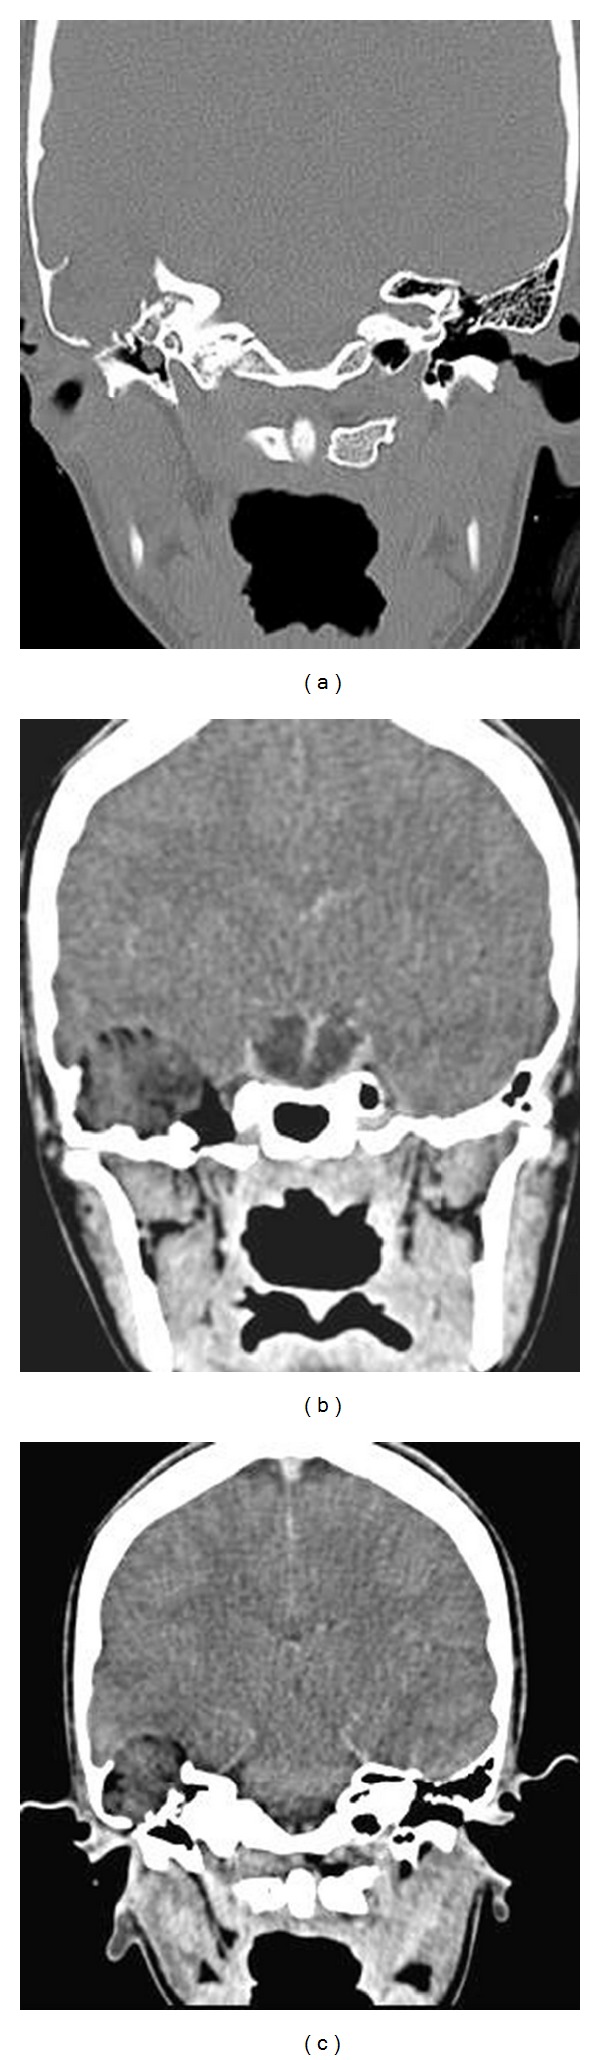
Computed tomography: coronal image, bone window (a) and soft tissue window with contrast (b and c) showing sclerotic contracted right mastoid temporal bone with most mastoid air cells being occupied by polypoidal expansile soft tissue densification lesion (heterogeneous, nonenhanced in contrasted soft tissue window image).
